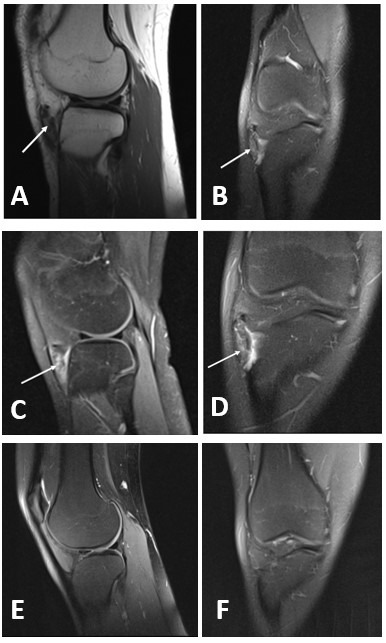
Preoperative and postoperative magnetic resonance images (MRI) of the right knee. (A) preoperative native MRI showing hemosiderin deposits in the area of the deep infrapatellar bursa (arrow) in a proton density and T2-weighted turbo spin-echo image in sagittal projection; (B) preoperative native MRI showing hemosiderin deposits in the area of the deep infrapatellar bursa (arrow) in a fat-suppressed proton-density-weighted turbo spin-echo image in coronal projection; (C) preoperative contrast-enhanced MRI showing proliferation of the synovium with signs of postcontrast imbibition, as well as hemosiderin deposits (arrow) in a T1-weighted spin-echo contrast-enhanced image in sagittal projection; (D) preoperative contrast-enhanced MRI showing proliferation of the synovium with signs of postcontrast imbibition, as well as hemosiderin deposits (arrow) in a fat-suppressed proton-density-weighted turbo spin-echo contrast-enhanced image in coronal projection; (E) postoperative MRI performed 18 months after surgery, showing no recurrence of the tumor in a proton-density-weighted turbo spin-echo image in sagittal projection; (F) postoperative MRI performed 18 months after surgery, showing no recurrence of the tumor in a proton-density-weighted blade fat-saturated image in coronal projection.
